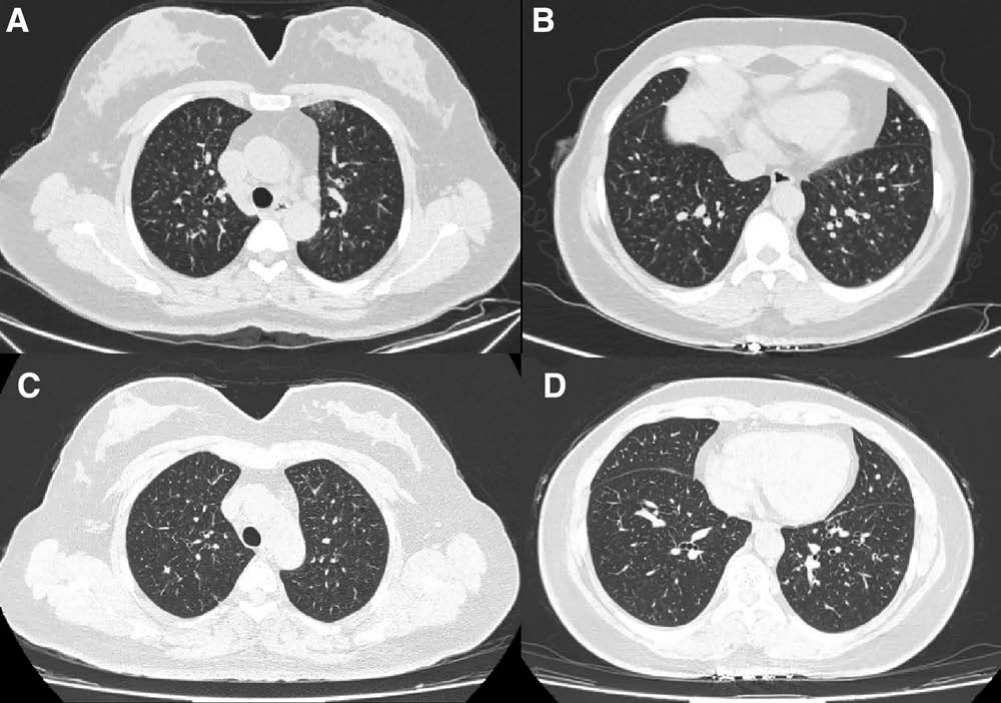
CT scan of the lungs: (A and B): At the initial visit: Bronchitis, double viral pneumonia and multiple inflammatory nodules in both lungs. (C and D): At the return visit: Remission of double pneumonia.
